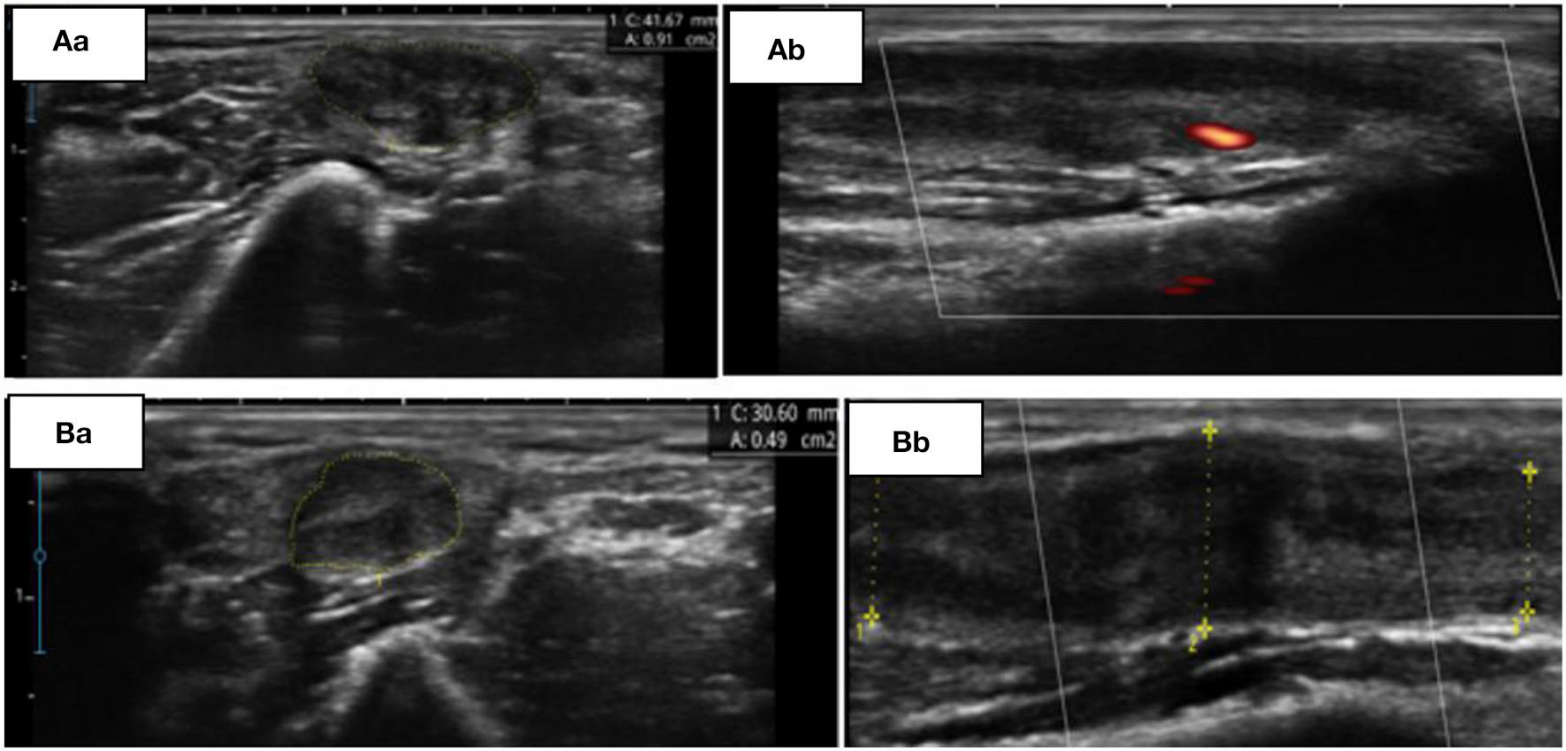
(A) Initial findings from ultrasonography (USG). Transverse (Aa) and longitudinal (Ab) view of the left ulnar nerve at the supraepicondylar region. (B) Control USG, 60 days later. Transverse (Ba) and longitudinal (Bb) view of the same nerve at the supraepicondylar region.
